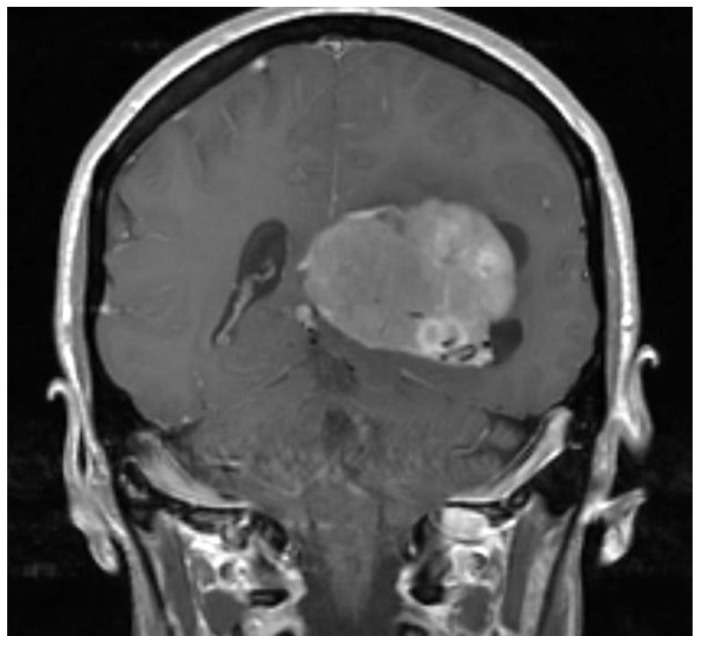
Pre-operative magnetic resonance imaging (MRI) demonstrates a large meningioma in the atrium of the left lateral ventricle.
